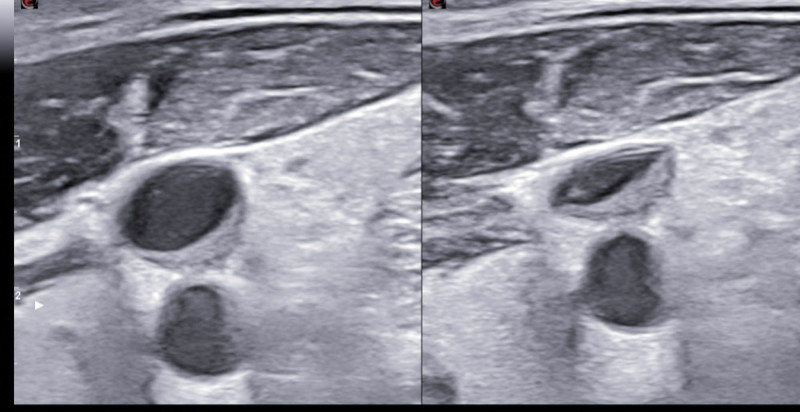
Ultrasonography 1-year follow-up images: short axis view of the left jugular vein (compression ultrasound CUS maneuver) and long axis view of the left jugular vein and subclavian vein, showing residual slight thrombosis only of the left jugular vein.
